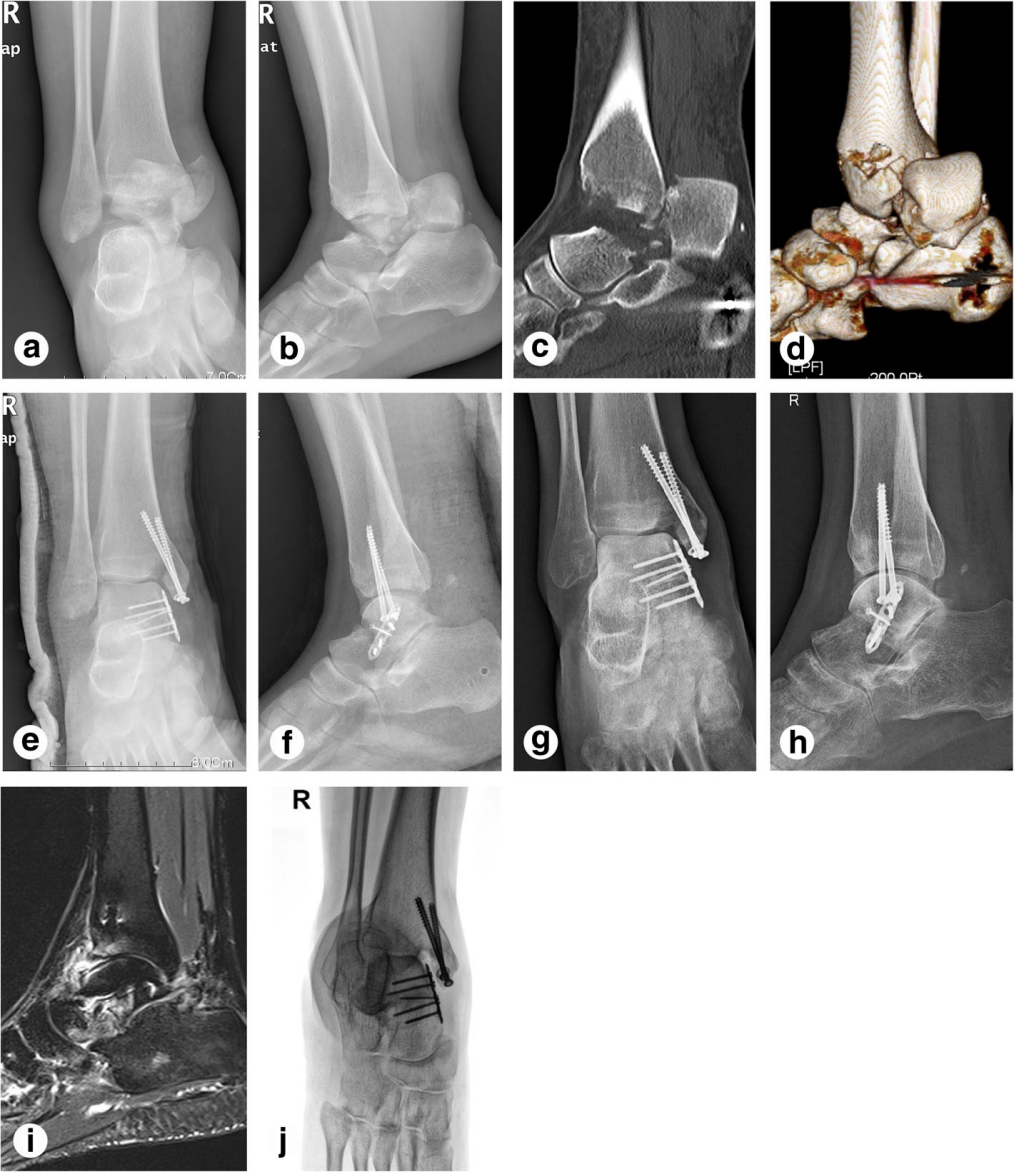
Examples of images obtained from case 18 (A 23-year-old female patient with a right ankle injury due to heavy object crushes). a–b Anterioposterior and lateral view of X-ray before operation. c–d CT and 3D reconstruction before operation. e–f X-ray at 7 days after operation. g–h Anterioposterior and lateral view of X-ray at 6 months follow-up. Subtalar arthritis was obviously seen. i MRI at 1 year follow-up. Talocrural and subtalar arthritis were obviously seen. j Canale view of X ray at 1 year follow-up
